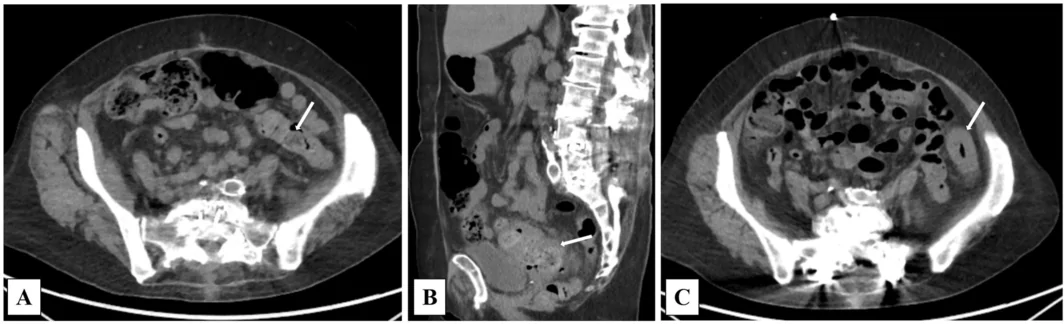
Non‐contrast CT of the abdomen and pelvis. (A) Transverse and (B) sagittal views obtained at the current presentation, and (C) transverse view obtained 5 months prior, demonstrating persistent mild wall thickening (white arrows) involving the distal transverse, descending, and sigmoid colon, with subtle adjacent pericolic fat stranding, findings suggestive of colitis. No lymphadenopathy is identified. Multiple colonic diverticula are incidentally noted without evidence of acute diverticulitis. Atheromatous calcifications of the abdominal aorta and its branches are also noted, without CT evidence of vascular occlusion or ischemic bowel.
